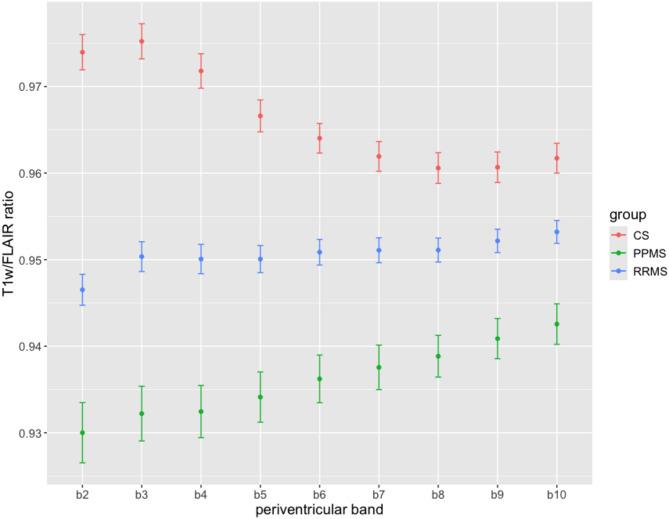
T1w/FLAIR ratios within periventricular bands. Mean T1w/FLAIR ratios of normal-appearing white matter in each periventricular band (band b2 to band b10) for control subjects (CS), patients with primary progressive MS (PPMS), and patients with relapsing-remitting MS (RRMS). Error bars represent standard errors
